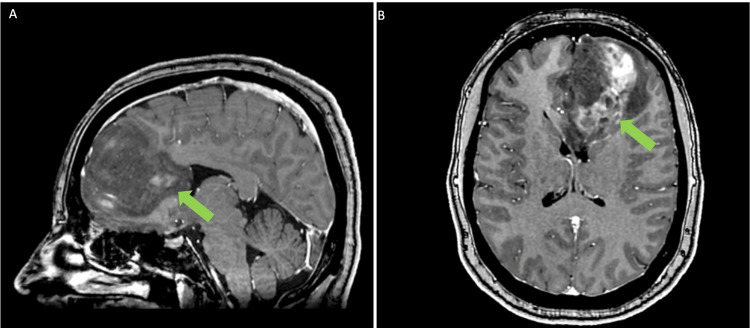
Preoperative Imaging A) Sagittal and B) Axial view of patient preoperative MRI. Location of frontal glioma indicated by green arrow.
